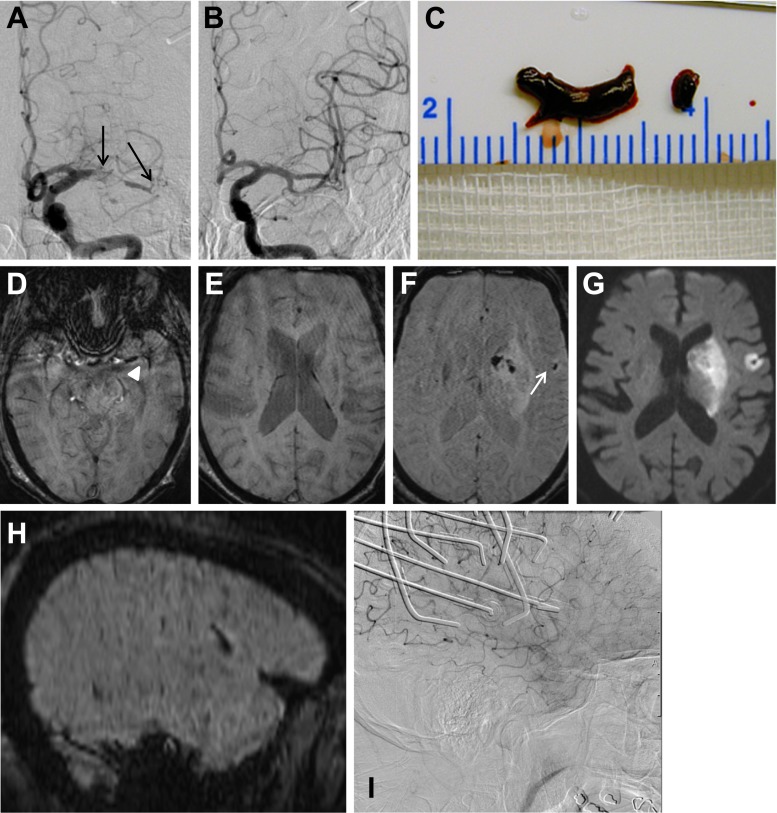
A 83-year-old man with right-sided hemiparesis (NIHSS score 21). Two separate thrombi in the left proximal M1 segment and the MCA bifurcation are seen on DSA (a, *black arrows*). Pre-interventional SWI shows thrombotic material in the proximal M1 segment and MCA bifurcation extending in an M2 branch (measuring 10 and 9 mm on SWI; d, *white arrowhead*) but no additional distal fragments (e). Two thrombus fragments were retrieved (c). Final biplane DSA control was rated as TICI 3 reperfusion (b). Post-interventional SWI detected one EE (f, *white arrow*) with an associated ischemic lesion on DWI (g). The sagittal reformatted projection of the SWI confirms the extracerebral location of the EE and its orientation along a suprasylvian MCA branch (h). Lateral DSA shows stagnating flow in the corresponding MCA branch with discretely diminished parenchymal blush during this phase of the DSA (i). (Artifacts are produced by simultaneous recording of NIRS)
